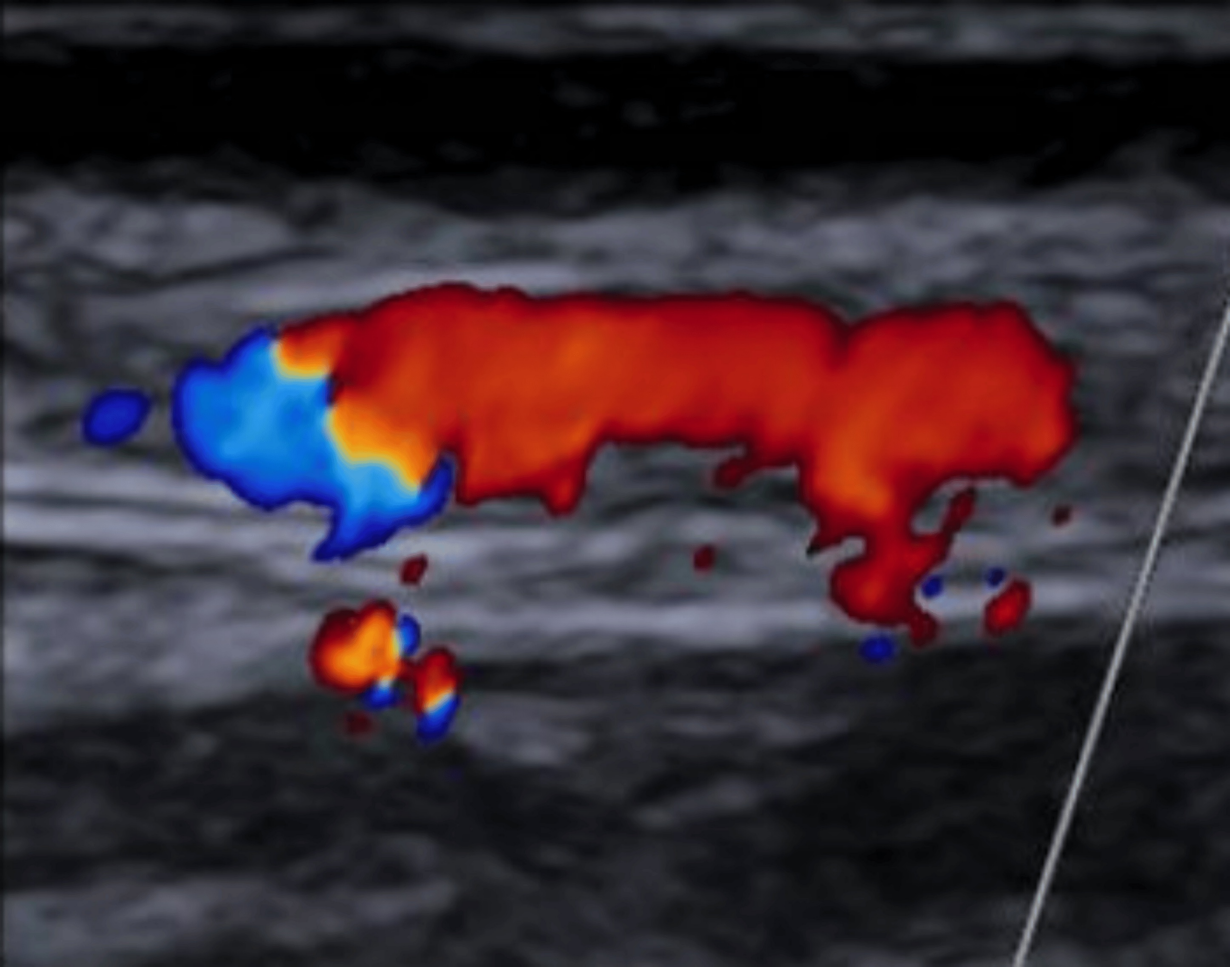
A longitudinal section of his repeat temporal artery colour doppler in July 2025 which shows a patent proximal frontal branch of the temporal artery with no evidence of vessel wall thickening or occluded lumen. The affected part however could not be visualised due to the biopsy.
